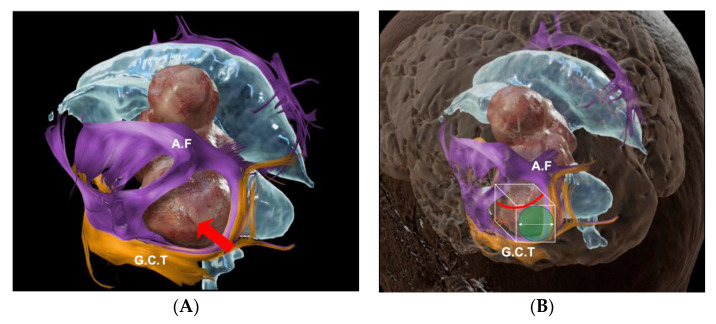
(A,B) show MRI rendering of a large intraventricular tumor (red arrow). Arcuate fasciculus (AF), geniculocalcarine tract (GTC) reconstruction helps in the planning stange for the resection of the tumor.
